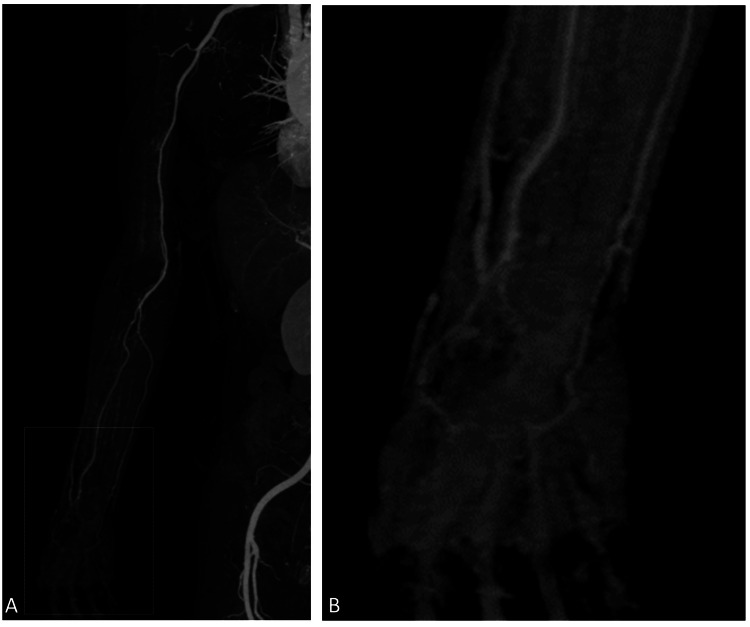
Contrast-enhanced CT at the time of emergency department (A) No occlusion of the puncture site, radial artery, or ulnar artery was patent. (B) The palmar arterial arch was partially poorly visualized but was not occluded entirely.
